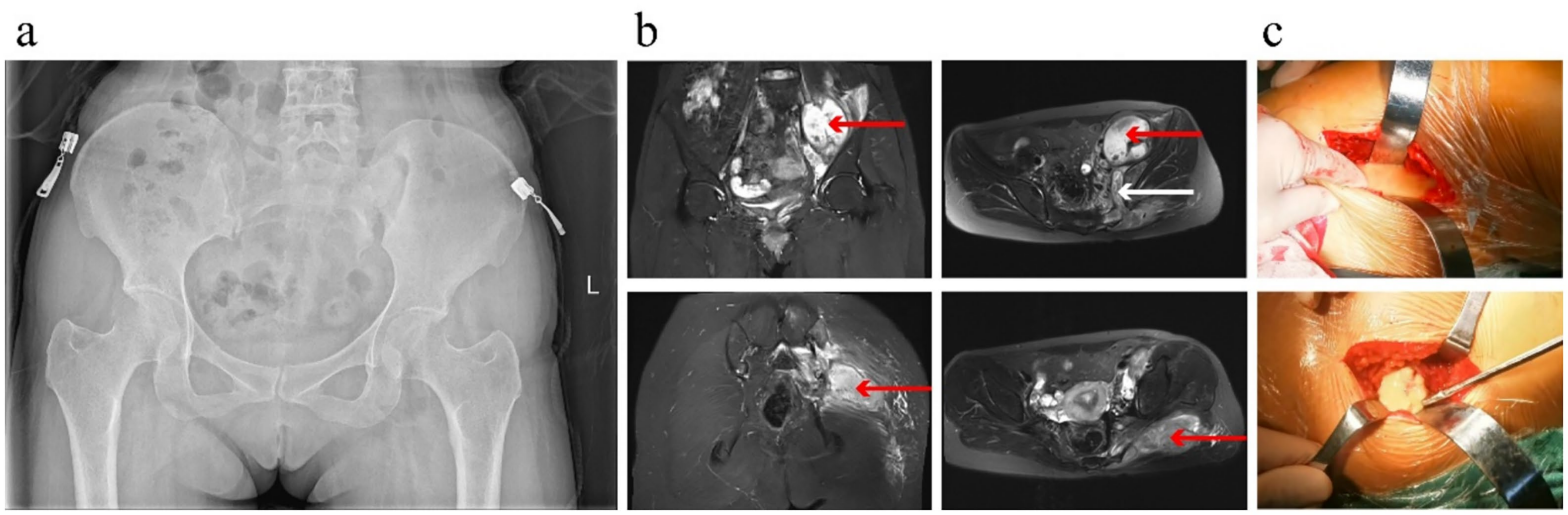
(a) X-ray examination shows no obvious abnormalities; (b) MRI reveals a mass measuring approximately 7.9 × 5.4 cm in the left iliac fossa and a 6.1 × 3.5 cm mass within the left gluteus maximus. The two lesions communicate through the left sacroiliac joint, with the iliac fossa abscess extending into the gluteal musculature. Abnormal signal changes are observed in the adjacent bone and soft tissues. Red arrows indicate the abscess lesions in the iliac fossa and gluteal region; black arrows indicate the communicating tract between the two regions; (c) Intraoperative findings show a large amount of yellow, turbid fluid mixed with caseous necrotic material within the iliac fossa and gluteal region.
